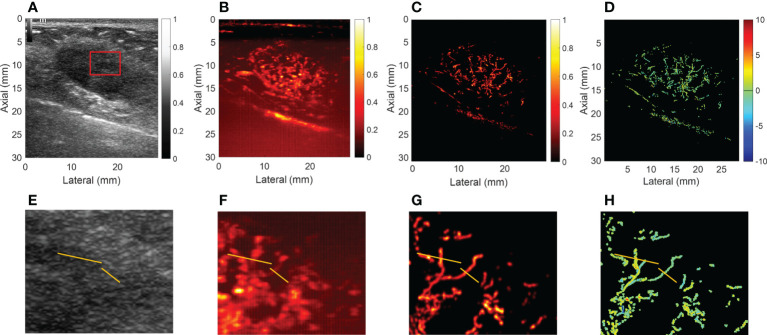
Ultrasound images of a representative benign breast mass. (A) B-mode image. (B) Contrast-enhanced ultrasound image. (C) Super-resolution image. (D) Super-resolved velocity image. (E–H) Shows the zoomed-in regions of (A–D) as the red box indicated in (A). Yellow lines in (E–H) highlight the resolution improvement among the images.
